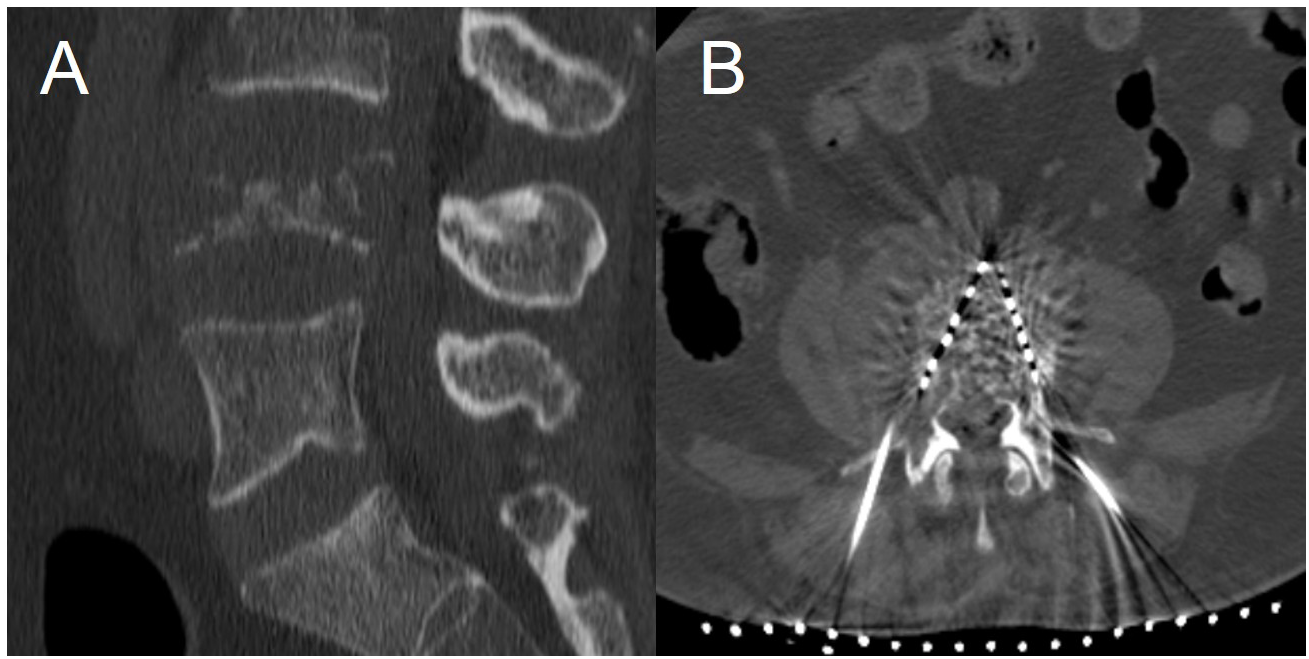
A 65-year-old male patient with lung cancer and L4 vertebrae bone metastasis. (A) The CT sagittal view shows that there is osteolytic bone destruction in the L4 vertebra. (B) A 125-I particle implantation procedure for the L4 vertebra was performed under CT guidance. The axial view showed that the 125-I particles were evenly distributed, and the patient experienced a significant immediate pain relief.
